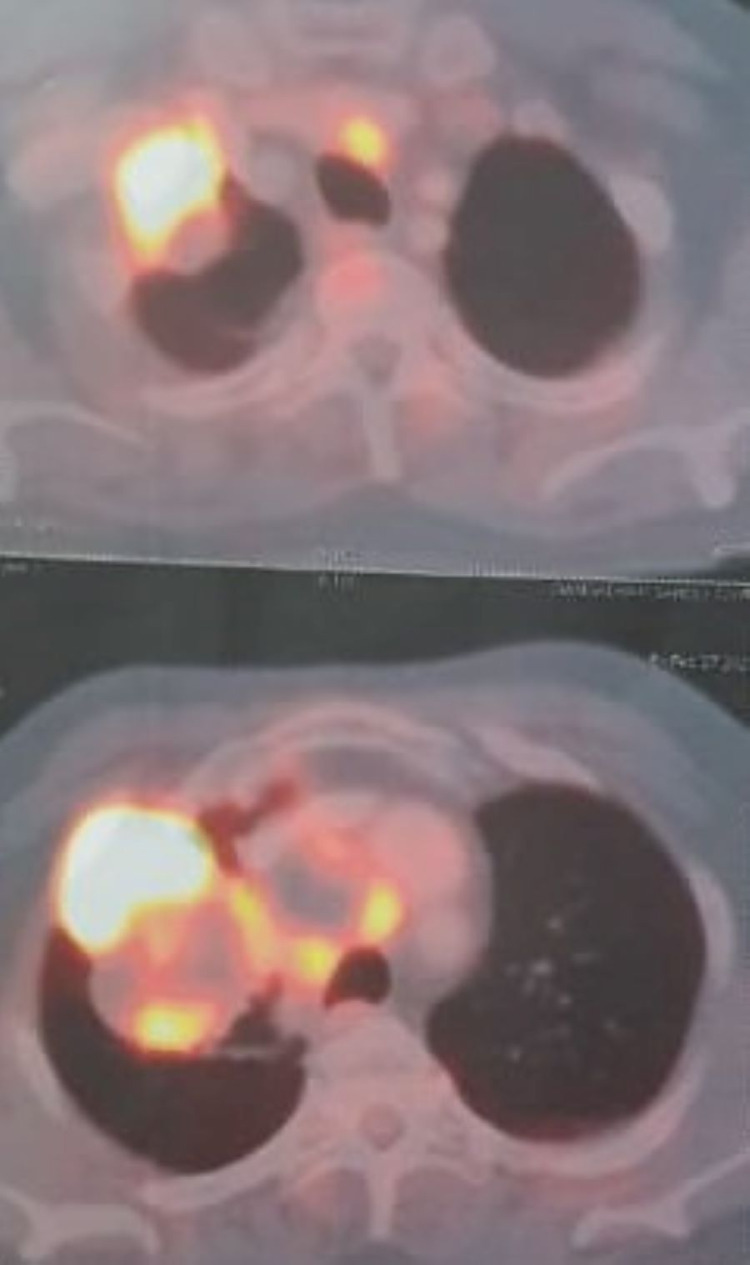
Re-evaluation PET-CT scan in February 2021 showing lesion (size 6.4 x 5.4 x 7.2 cm, SUVmax = 37.04) in upper lobe of right lung. PET-CT, positron emission tomography-computed tomography; SUVmax, maximum standardized uptake value.
